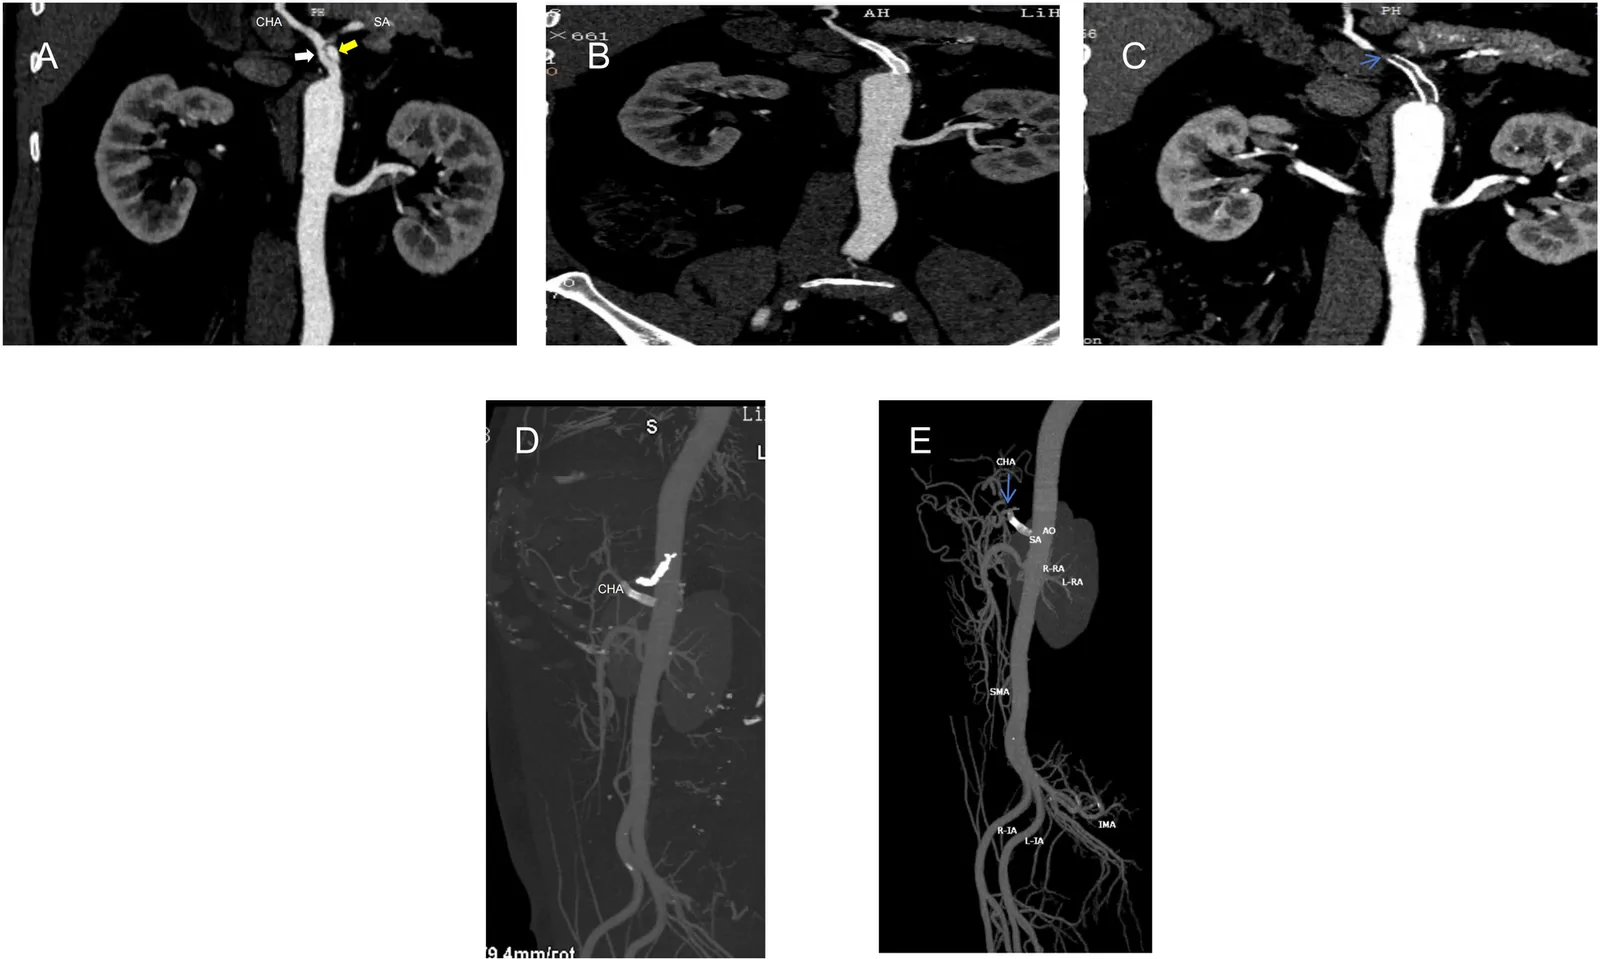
Representative case of stent occlusion after endovascular intervention for spontaneous isolated celiac artery dissection. (A) Baseline CTA (CPR) showing true lumen stenosis with false lumen (FL) compressing the true lumen (TL). (B) CTA (CPR) at 6-month follow-up demonstrating sustained stent patency. (C) CTA (CPR) at 2-year follow-up demonstrating complete stent occlusion (arrowhead). (D, E) 3D VR reconstructions at 6-month and 2-year follow-ups. At 6 months (D), the stent is patent with good flow into CHA. At 2 years (E), stent occlusion (arrowhead) with collateral circulation via pancreaticoduodenal arcades maintains visceral perfusion, explaining the asymptomatic status. Mechaism of occlusion: Stent occlusion was likely attributable to incomplete endothelialization of the covered stent, local hemodynamic disturbances, and possible clopidogrel resistance. The patient remained asymptomatic due to robust collateral circulation. AO, aorta; CHA, common hepatic artery; SA, splenic artery; SMA, superior mesenteric artery; TL (white arrowhead), true lumen; FL (yellow arrow), false lumen.
